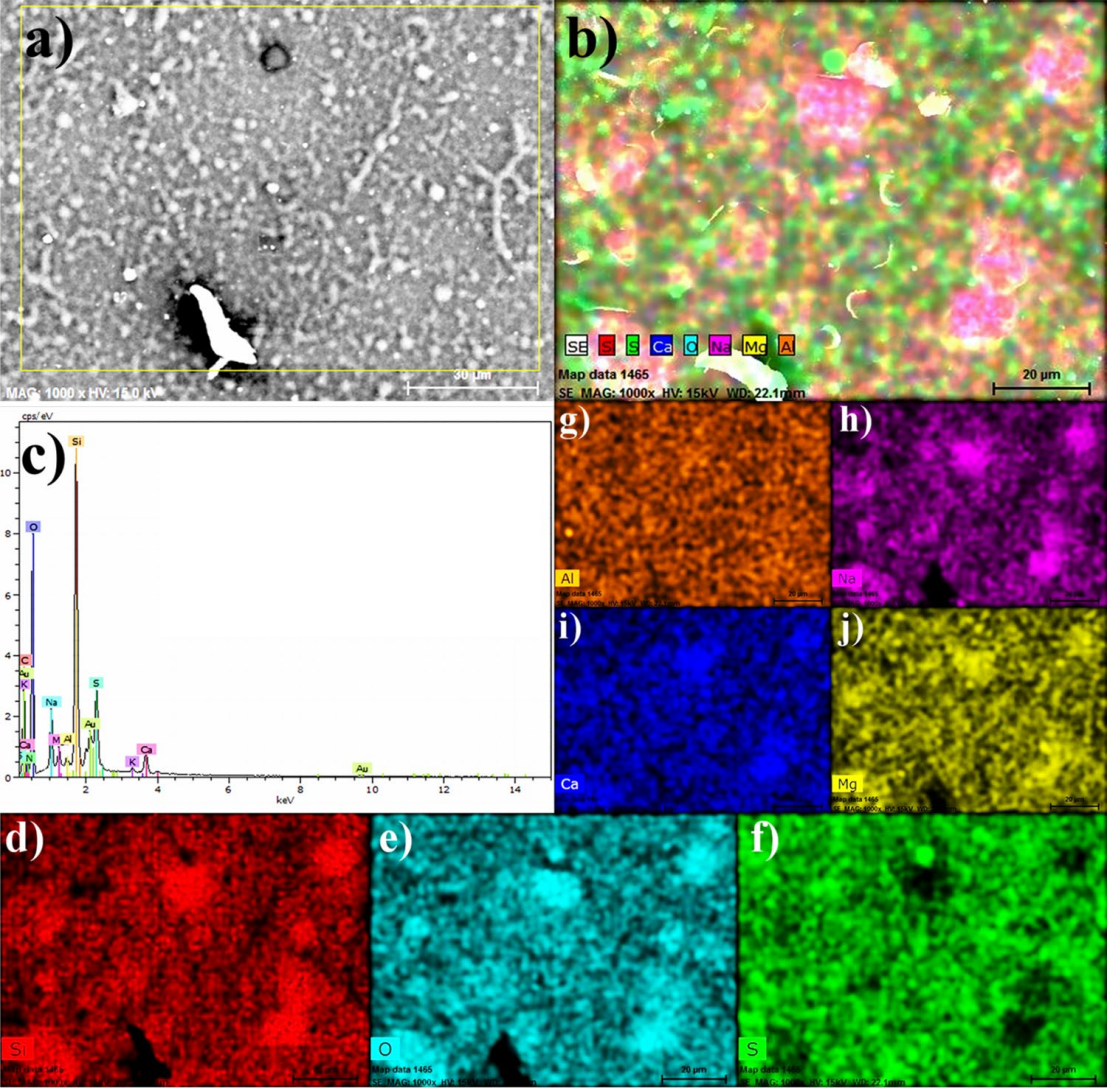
SEM and EDS analyses of silica deposited by APPJ and an ultrasonic fog of TEOS on a samarium compound coating by APPJ on aluminum 3003.
